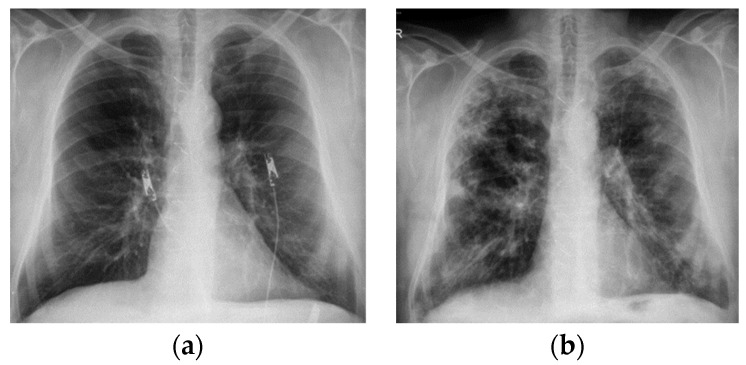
Chest X-ray—2019—Case 1. July 2019: no signs of interstitial damage; October 2019: reticular opacities associated with ground glass opacities with heterogeneous distribution, located subpleural especially. (a) July 2019; (b) October 2019.
